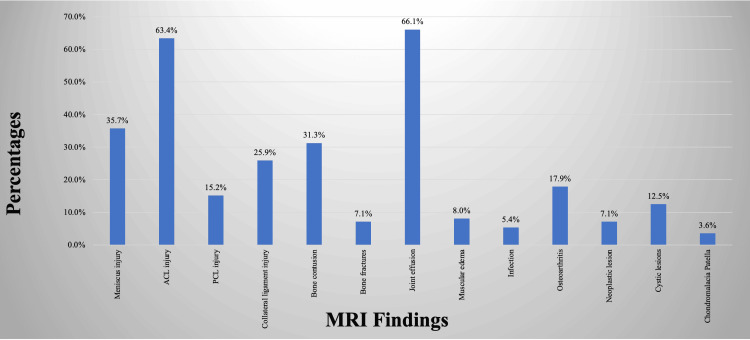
MRI findings in the study population and their frequency
